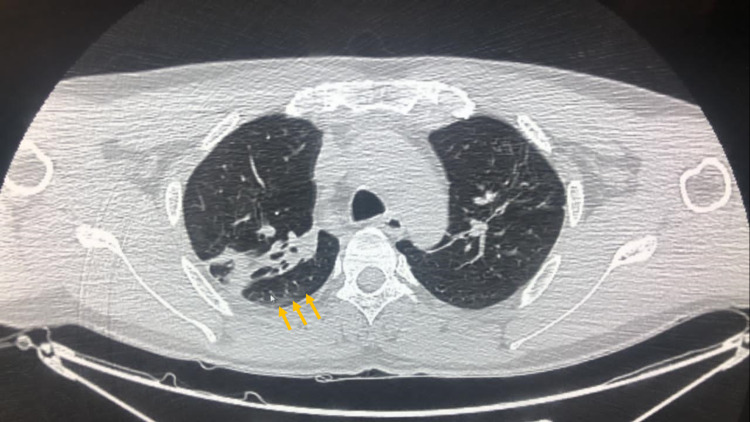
Chest CT-Scan Chest CT-Scan showing asymmetric bilateral ground-glass opacities estimated between 5-10% (yellow arrows)
